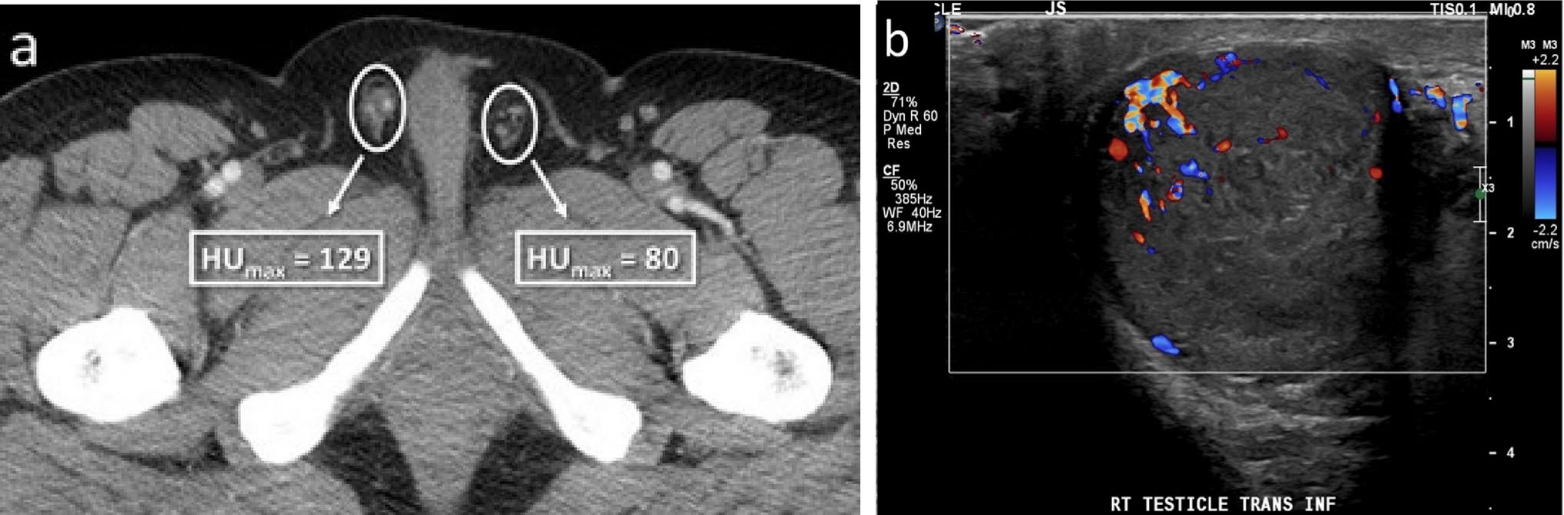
38-year-old patient with vague right-sided abdominal pain and testicular swelling. Quantitative analysis showed 62% greater right-sided differential enhancement (a). All readers reported an abnormal right cord with high confidence (5 on a 5-point Likert scale) with asymmetric enhancement and inflammatory change visible at CECT. Color Doppler ultrasound showed right testicular embryonal cell carcinoma (b). Cord abnormalities were not appreciated at the prospective CT read
